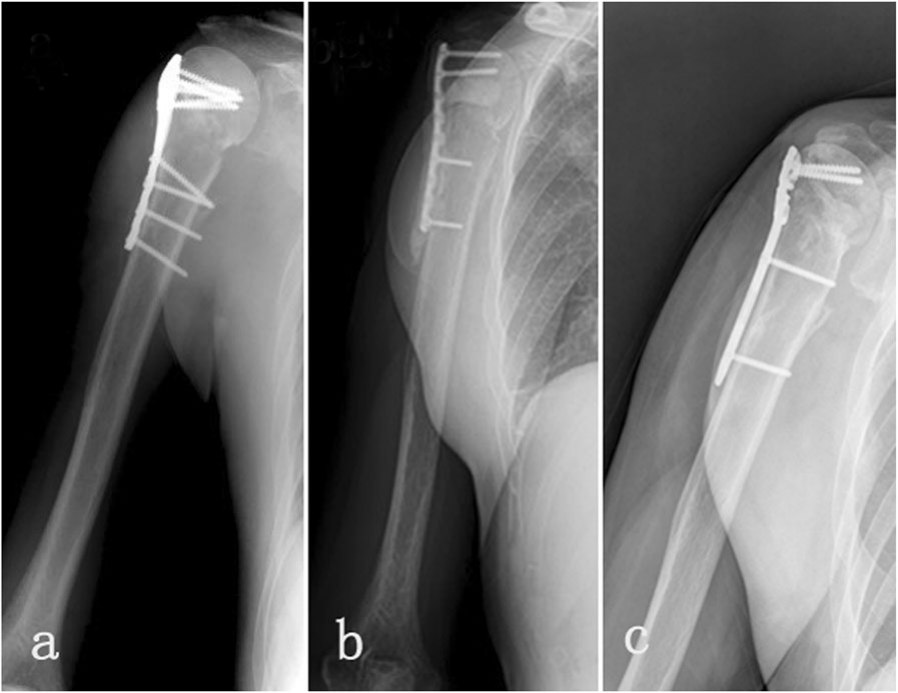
Imaging results of a patient with humeral osteomyelitis (Patient 13) a X-ray of a patient with post-traumatic osteomyelitis of the proximal humerus. b Post-debridement X-ray with an antibiotic-coated cement spacer and a coated plate for internal fixation. c Bone healing state 2 years after bone grafting
